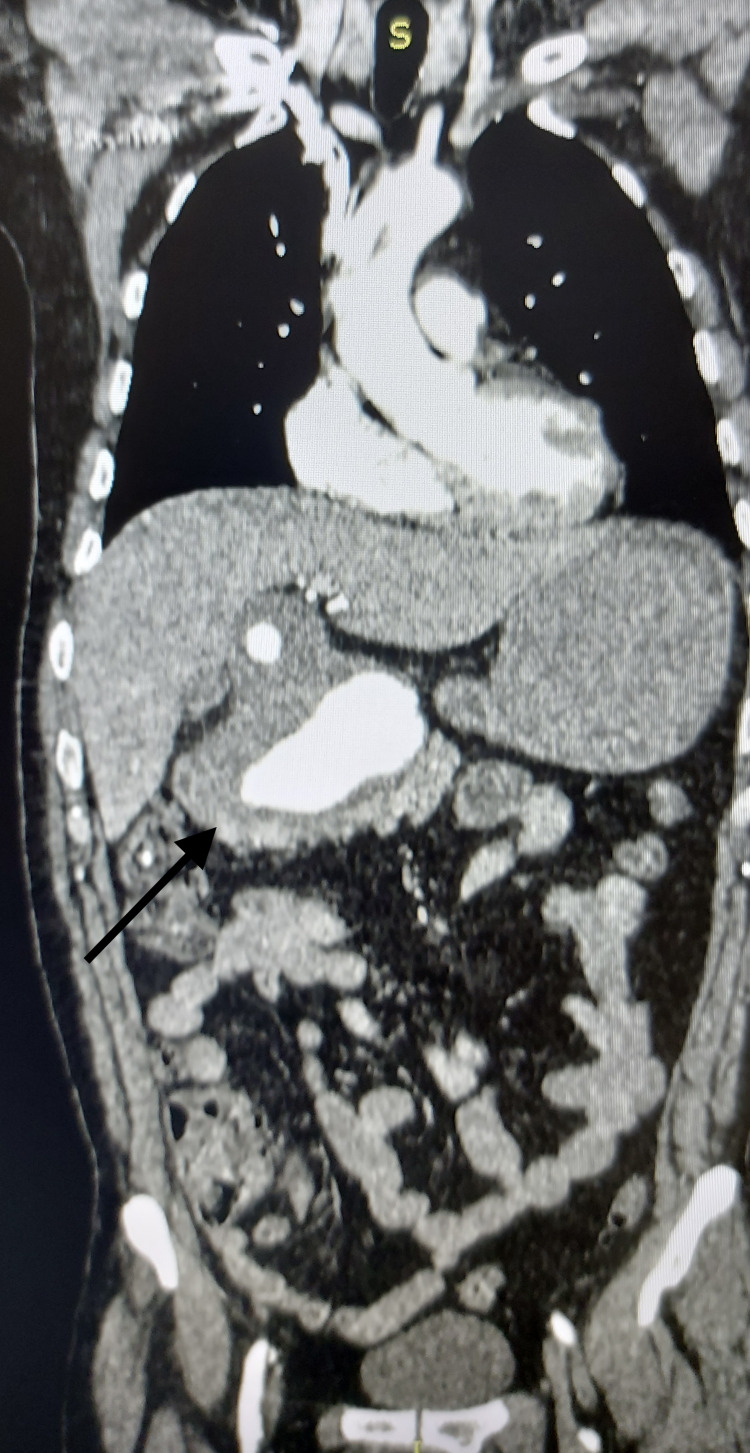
Contrast-enhanced CT coronal view of the giant hepatic artery (black arrow). CT: computed tomography
